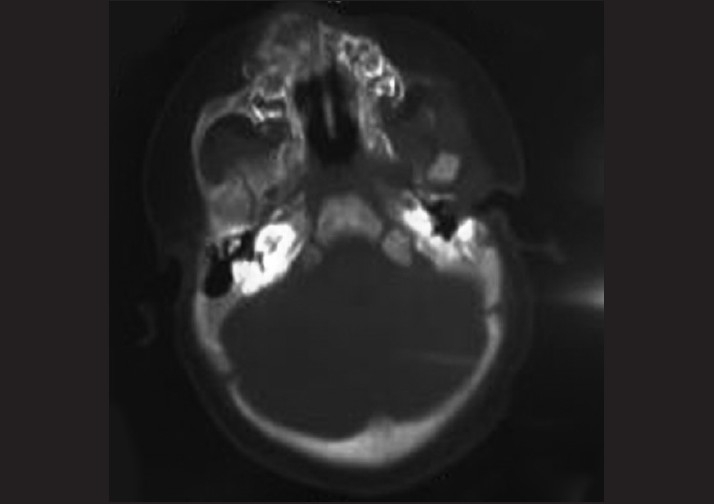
Computed tomography scan showing lobed extensive tumor of the anterior maxilla and central hypodense area
